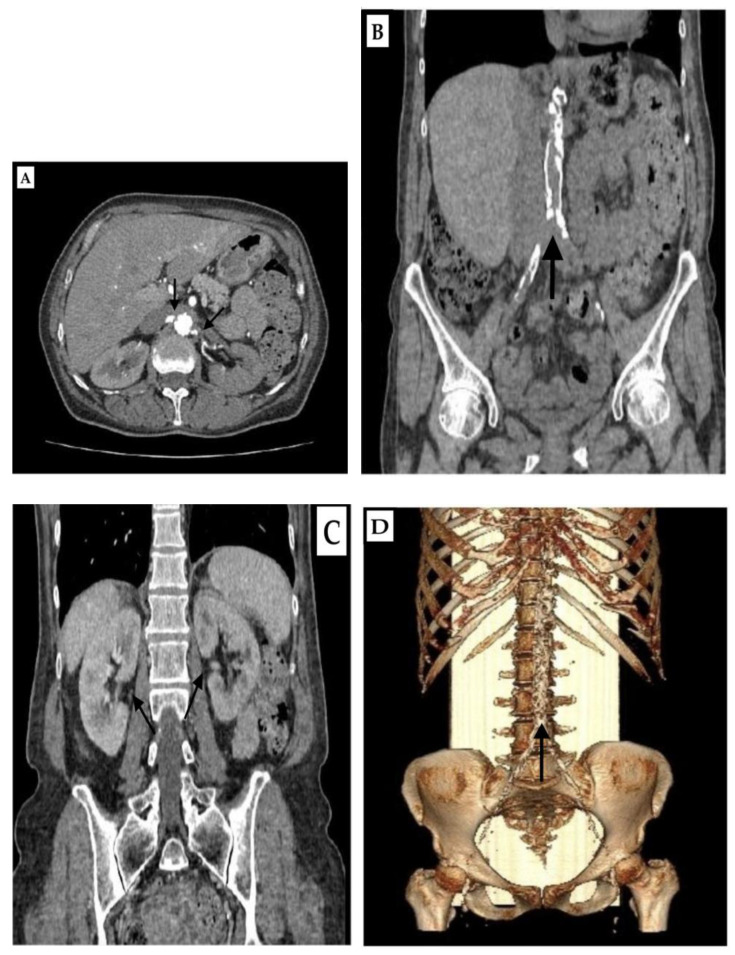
(A–D) Computed tomography angiography of the abdomen. Axial section, arterial phase, and narrowed lumen at the level of origin of renal arteries (A). Native shot, coronal section, and highly calcified aorta (B). Coronal section, porto-venous phase, and both kidneys post-contrast-opacified (C). Volume rendering (VR) view of a very pronounced calcified aorta (D). (Black arrows).
